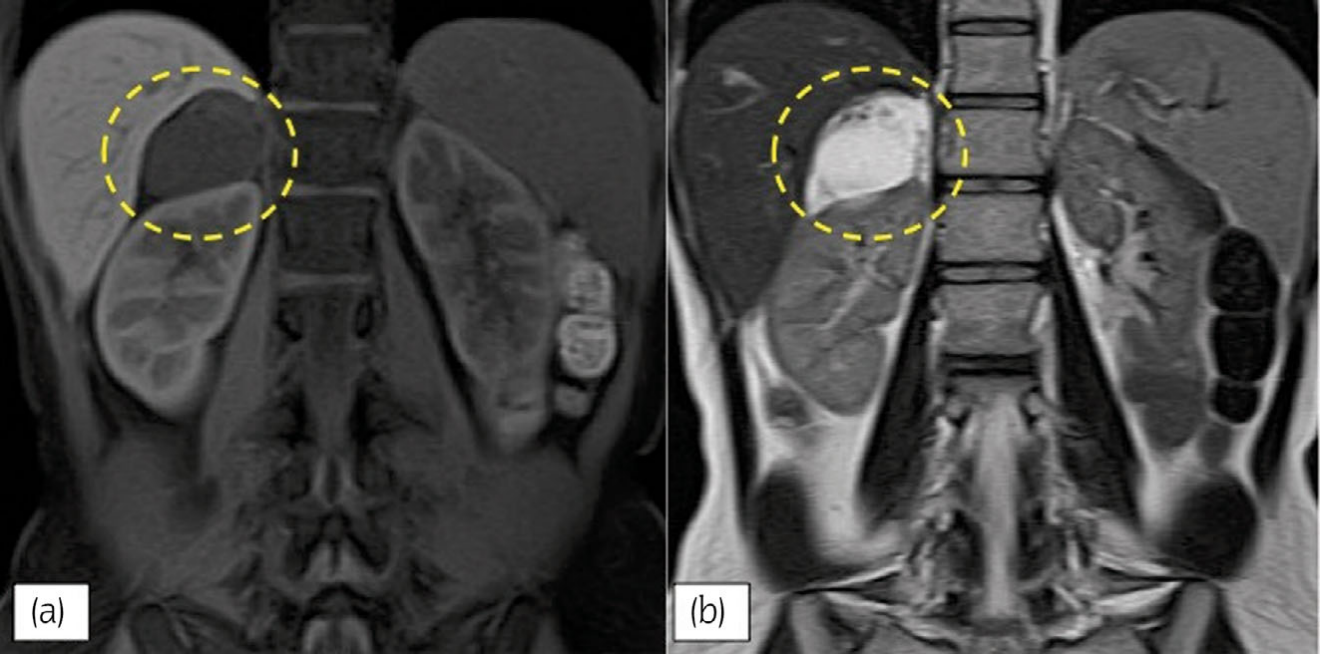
Abdominal MRI, coronal section. (a) T1 weighted image, showing hypointense adrenal tumor, encircled. (b) T1 weighted image, showing hyperintense adrenal tumor, encircled.
